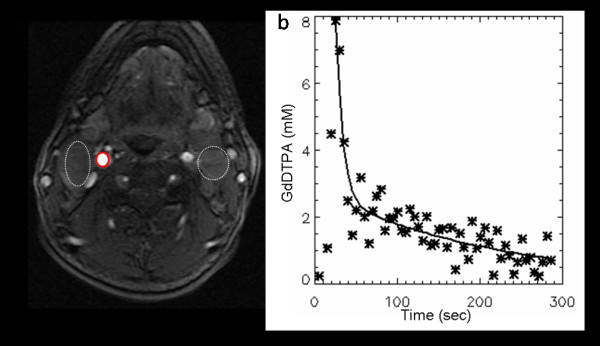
(a) A post-contrast axial MR image from a head and neck cancer patient extracted from the DCE-MRI scan showing bilateral nodal metastases (dashed white lines) in the neck and the right carotid artery (solid red line). (b) An arterial input function (AIF) plot (plasma Gd-DTPA concentration time course). The data points were measured from the region of interest placed within the right carotid artery. The AIF wash-out phase was fitted with a bi-exponential decay function.
